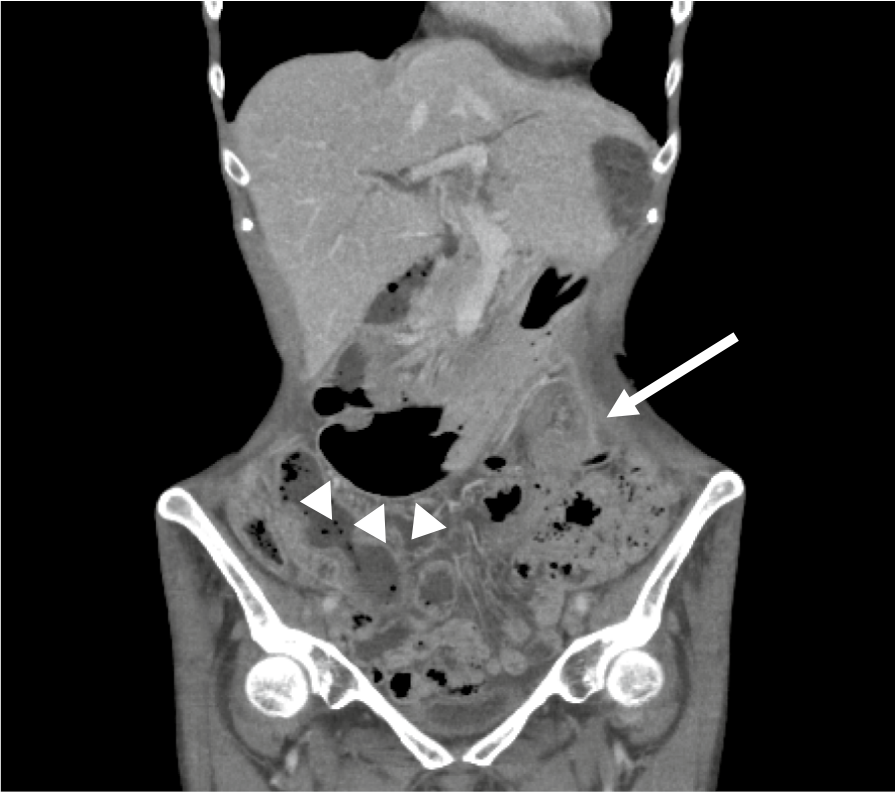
CT showed the position of the stomach after laparoscopic gastropexy was to the right of the lifted sigmoid colon. A white arrow and arrowheads point to the sigmoid colostomy and the stomach, respectively
